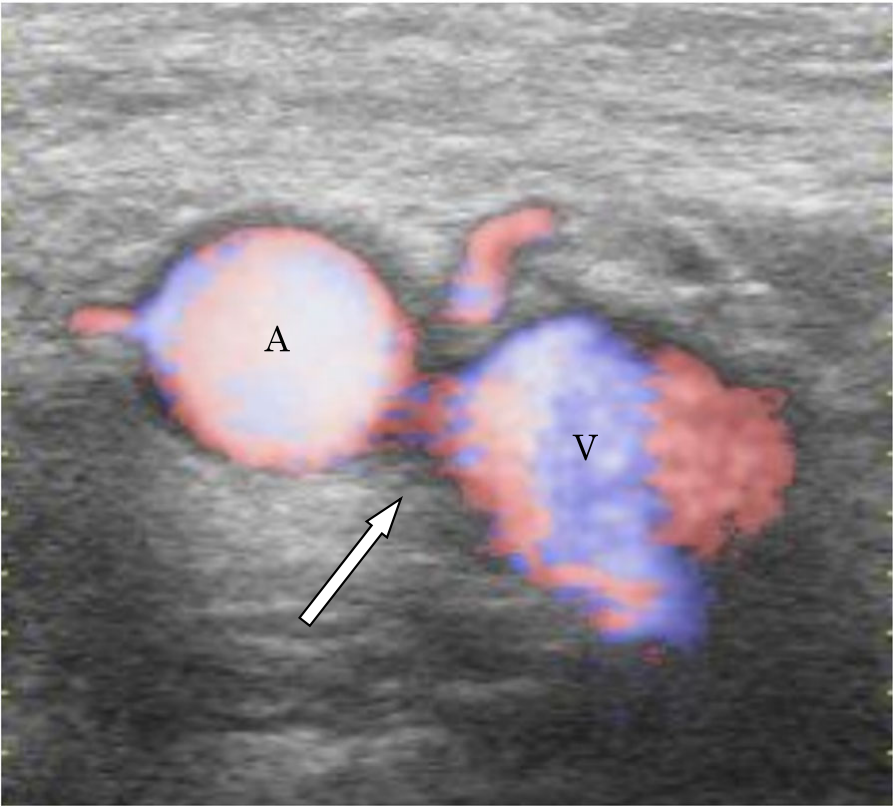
Color Doppler ultrasound findings of an arteriovenous fistula between the right femoral artery and the right femoral vein. A femoral artery, V femoral vein, arrow fistula
